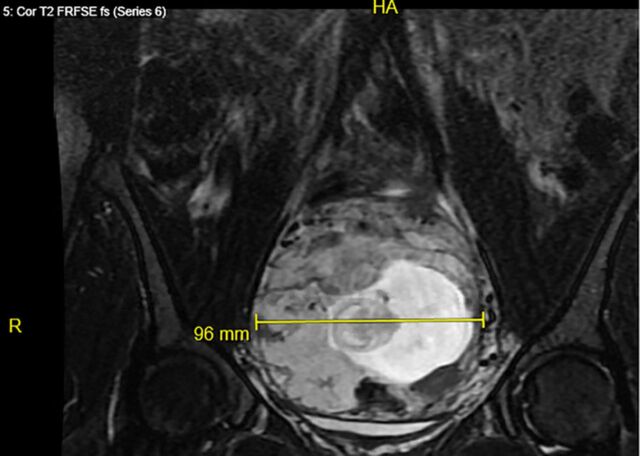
Coronal pelvic magnetic resonance imaging demonstrating the cervical ectopic pregnancy with measurement of the gestational tissue mass (96 mm).
